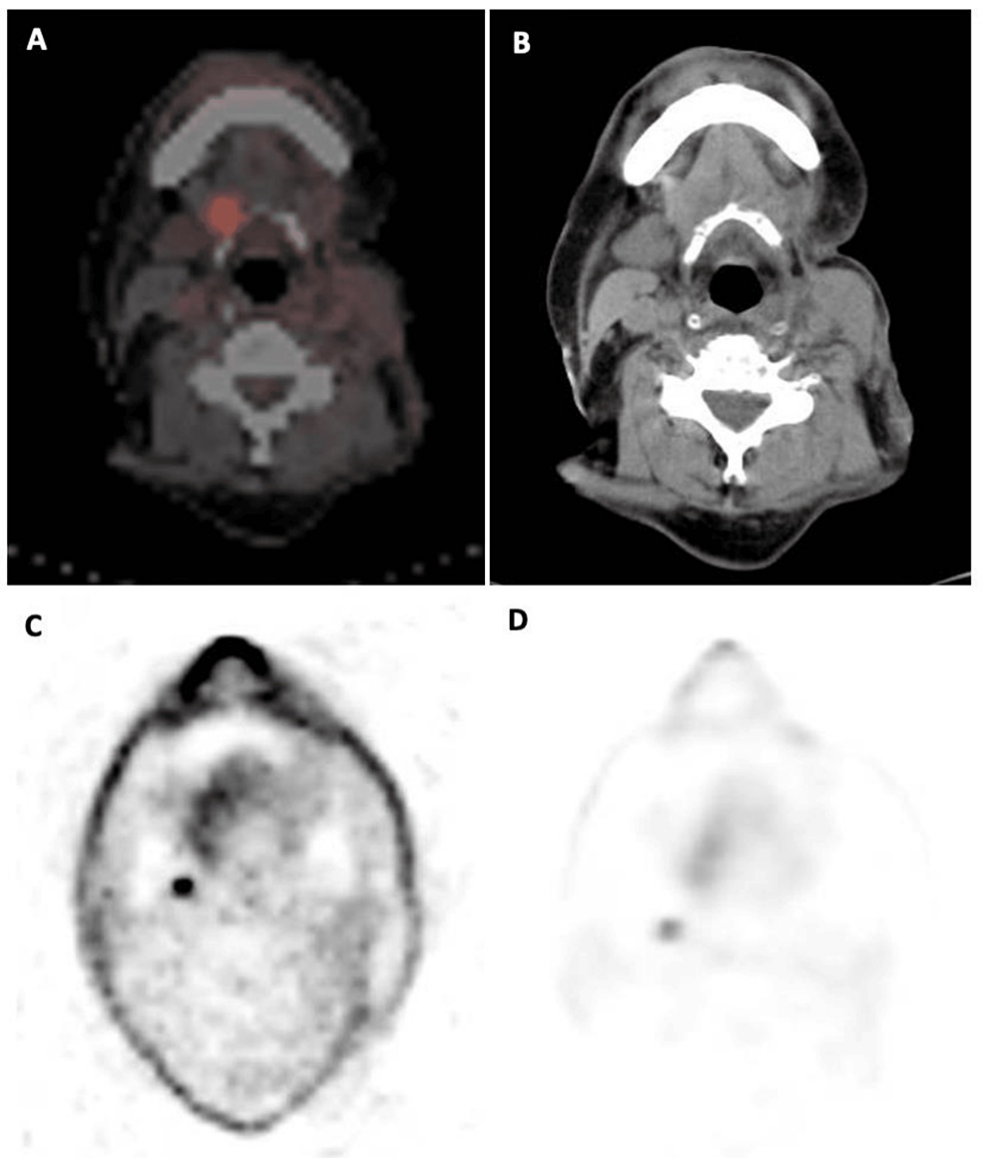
PET-CT showing an increased metabolic activity rate A and B: in left submandibular adenopathy; C and D: in left cervical adenopathy PET: Positron Emission Tomography. CT: Computed Tomography
